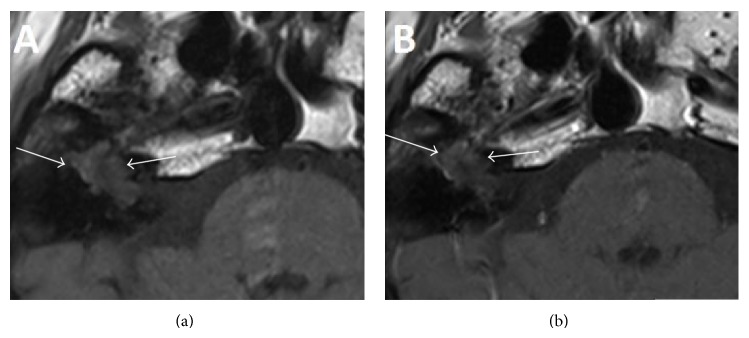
Pre- (a) and postcontrast (b) T1 weighted axial MRI imaging showing a largely isointense signal abnormality at the site of the mass seen on CT. There is no significant enhancement (*white arrows*).
